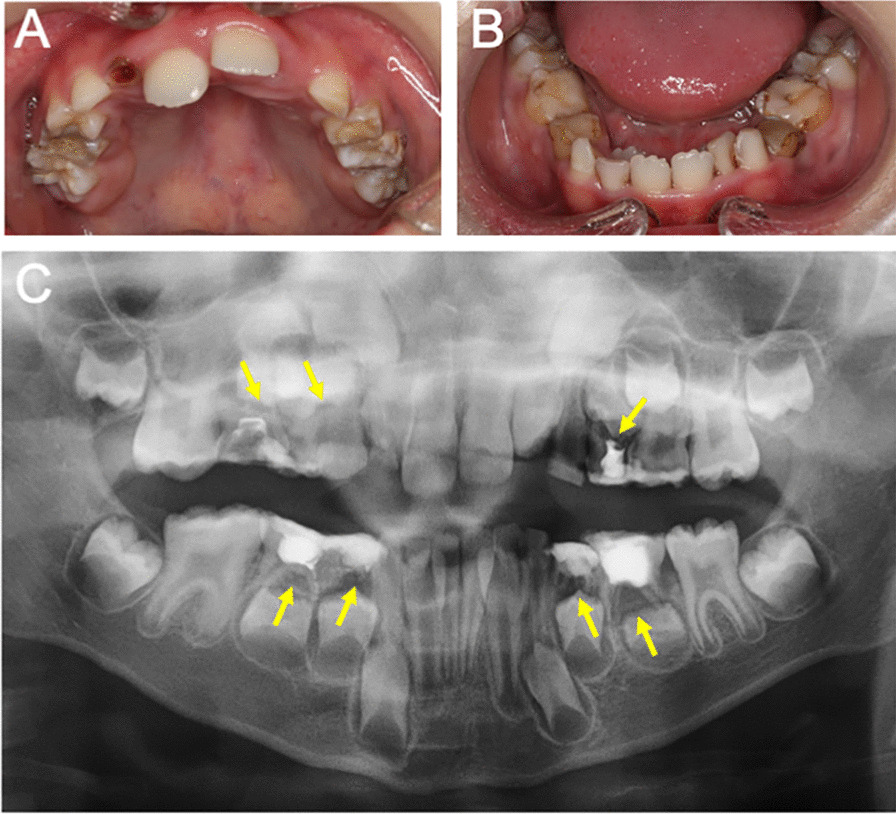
Clinical and radiographic findings of the patient at the initial visit. Intraoral photograph (A and B) and panoramic radiograph (C). Yellow arrows indicate apical periodontitis
